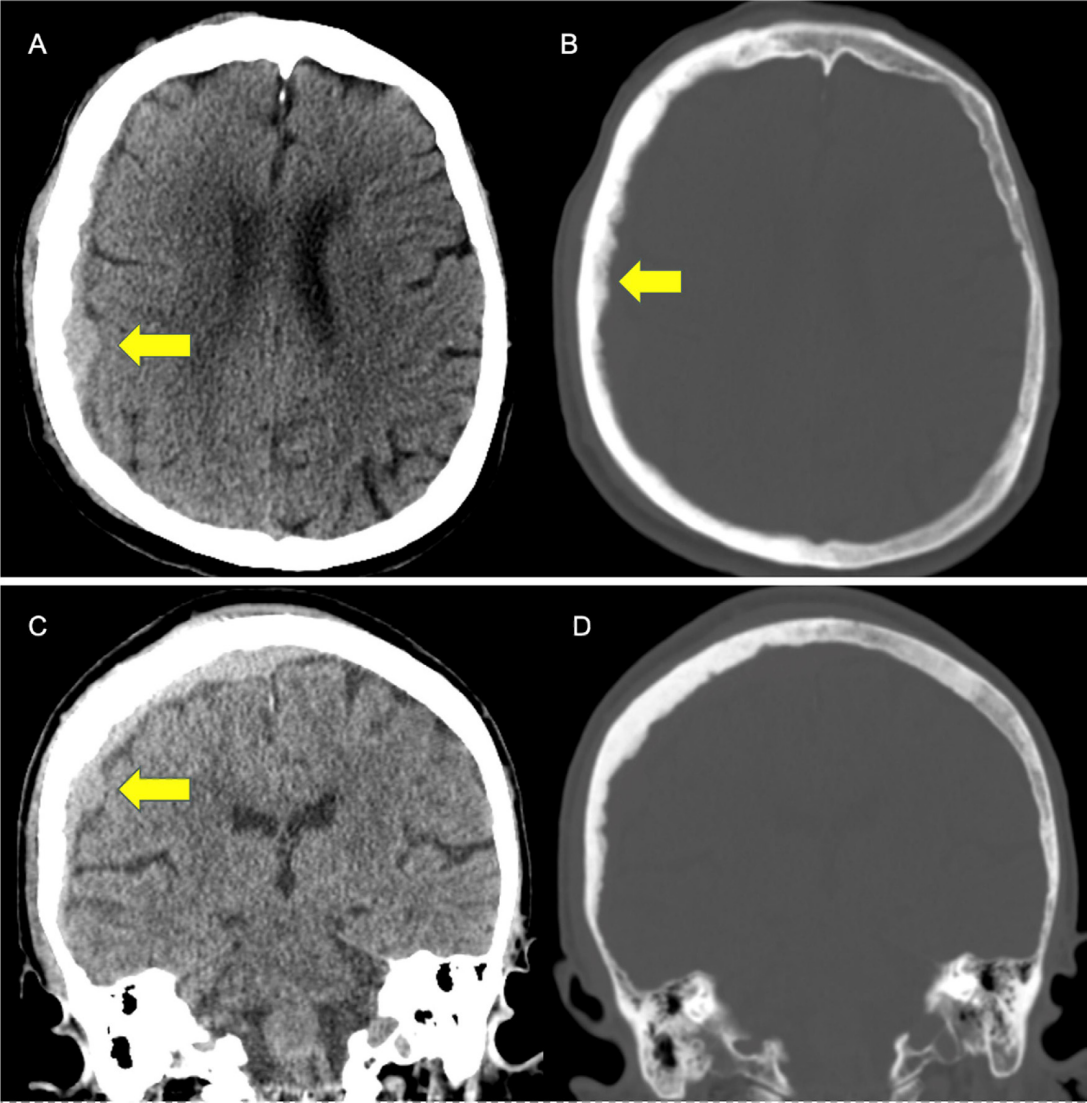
Computed tomography images of the head, axial, and coronal views. (A and C) Isodense subdural collection on the right (yellow arrow). (B and D) Bone view showing a hyperdense right skull when compared to the left along with subtle, hypodense portion near the inner cortex (yellow arrow).
